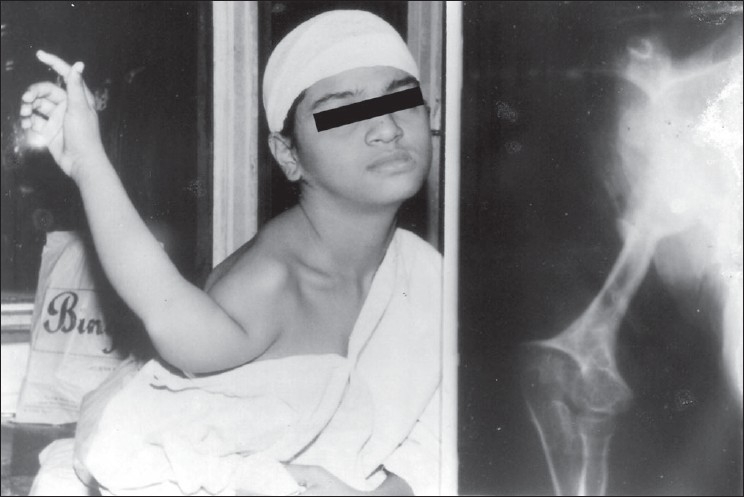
Preoperative clinical picture and roentgenogram (anteroposterior view) of humerus showing the deformity
